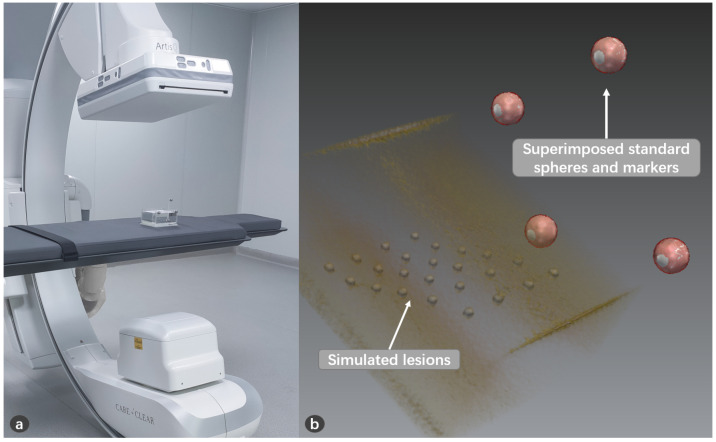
The patient registration. (a) CT scan the patient together with sphere markers; (b) CT image registered with sphere markers.
